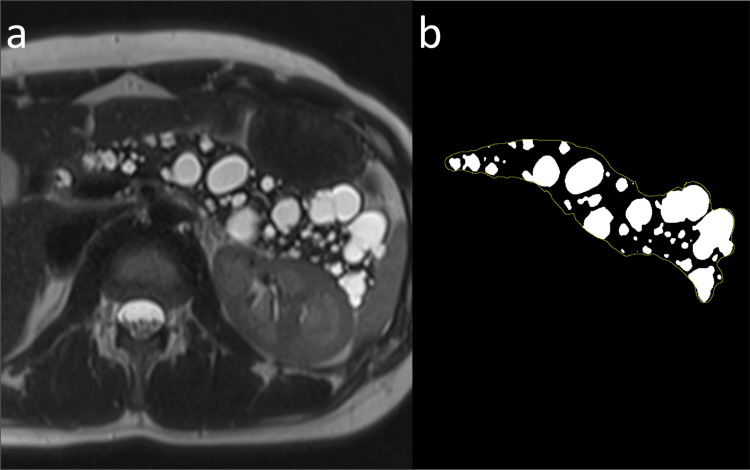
Measurement of pancreatic cysts in a 25-year-old woman without diabetes mellitus or high glycated hemoglobin level (a) An axial heavily T2-weighted magnetic resonance image that maximized the total area of the pancreatic body and tail is selected for each patient. (b) The image is changed to a black and white display according to a cut-off value. The cut-off value is adjusted such that the pancreatic cysts appear white and the regions without cysts appear black. The percentage of pancreatic cysts is 48.1%.
